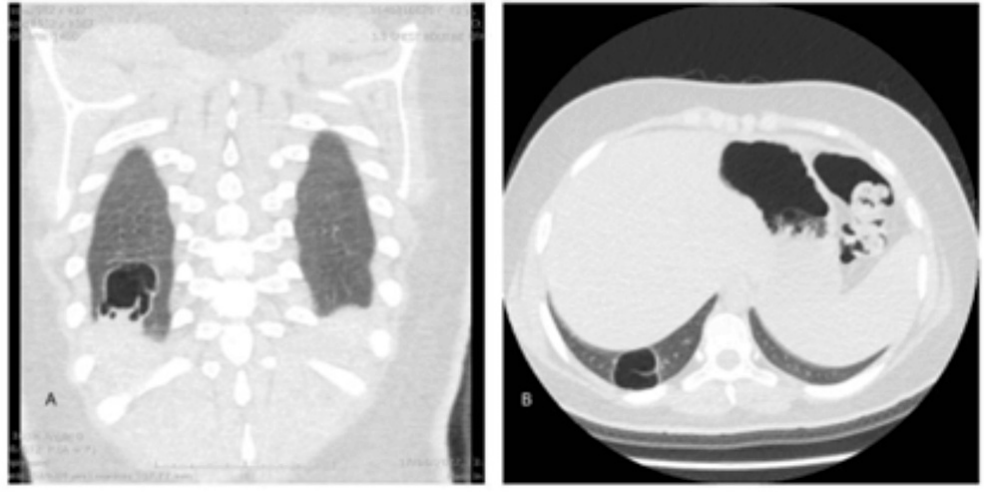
Chest CT showing a thick-walled large cavity in the right lower lobe with normal surrounding lung tissue.
